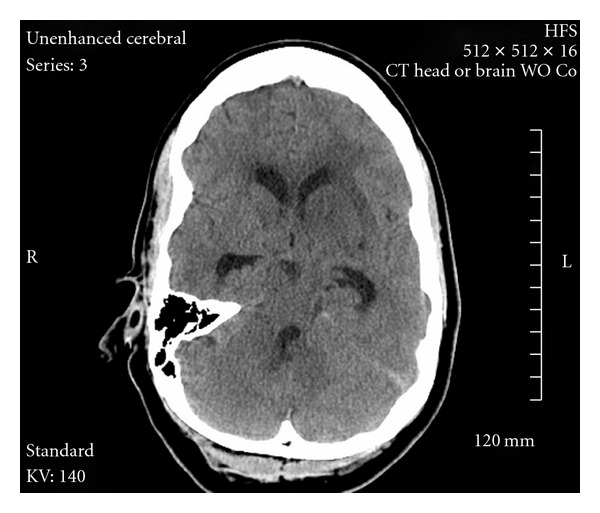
Noncontrast CT scan after 24 hours of intravenous Heparin.
